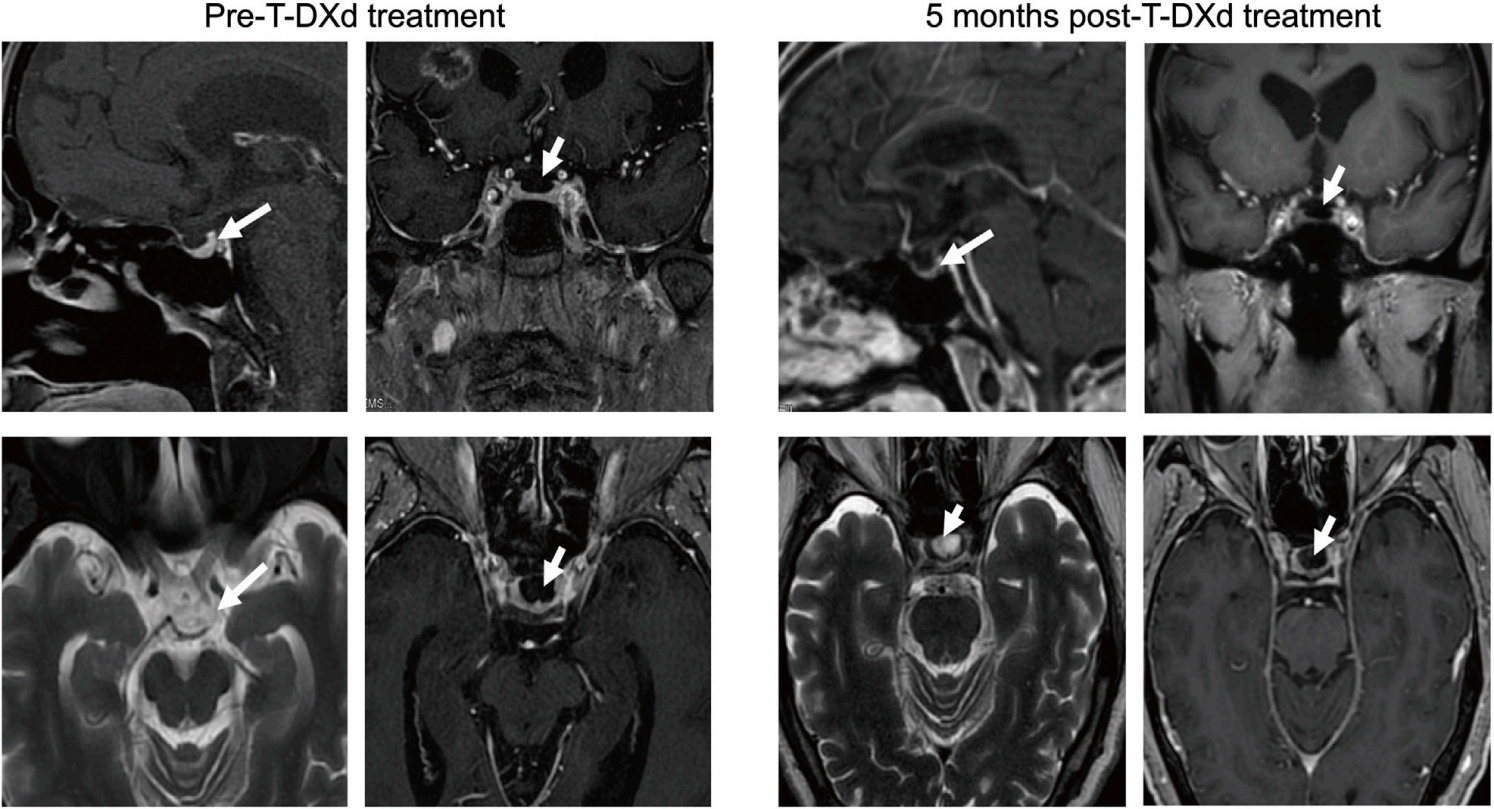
The patient’s cranial magnetic resonance imaging showed no improvement in empty sella turcica after Trastuzumab Deruxtecan treatment. The pituitary gland volume is reduced to a concave shape, and the empty sella turcica is present (white arrows).
